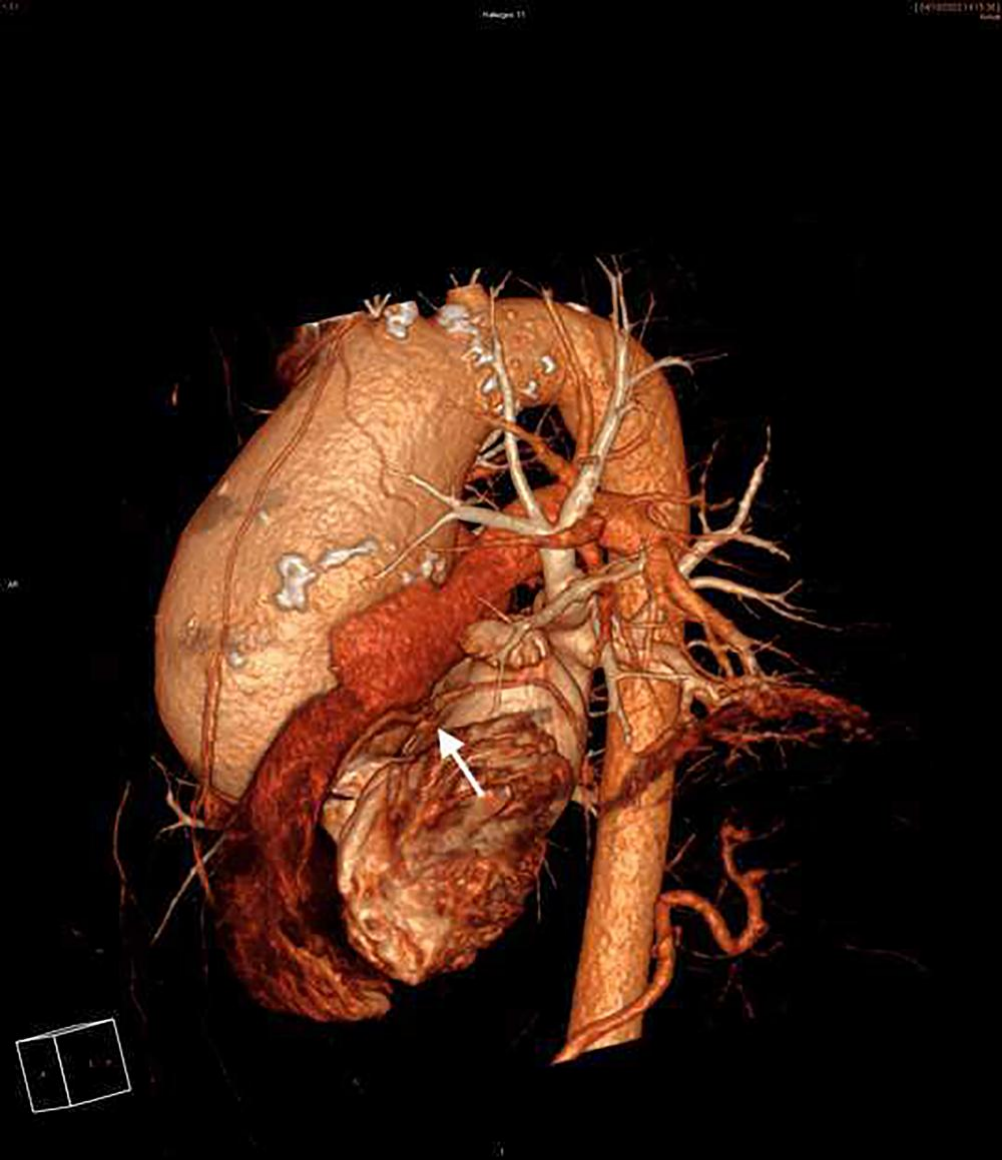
3D CT angiography reconstruction: giant aortic aneurysm and LCA (arrow).
